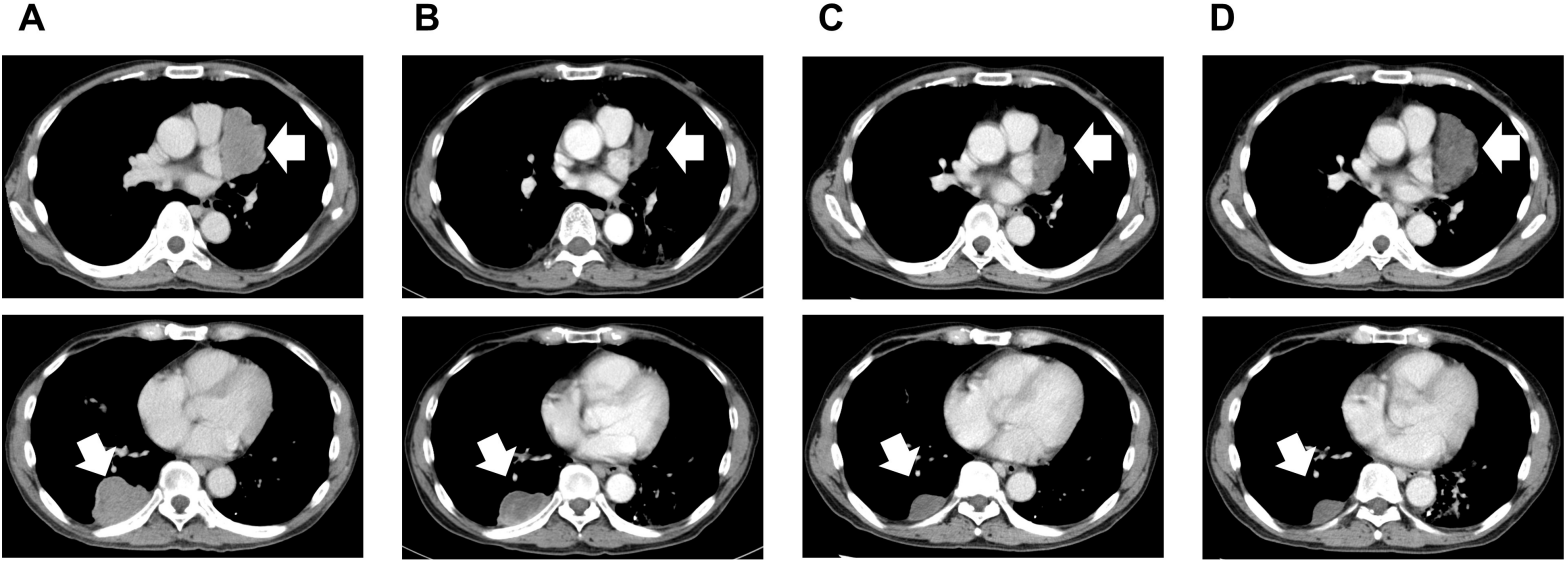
Computed tomography of metastatic lesions in the thoracic cavity before and during everolimus administration. Contrast-enhanced computed tomographic images at (A) the initiation of everolimus treatment and at 4 months (B), 1 year (C), and 2 years (D) after treatment initiation. Arrows indicate the tumor lesions.
